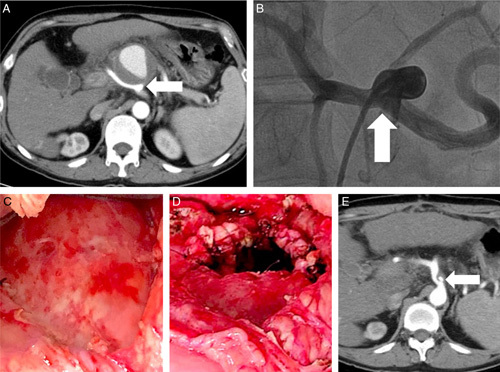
Celiac trunk false aneurysm (A) CT revealed an aneurysm from the celiac trunk (arrow). (B) The angiography showed a false aneurysm about 6 cm in diameter arising from the bifurcation of the celiac trunk(arrow). (C) The false aneurysm was about 7 cm in diameter, and challenging to control the feeding vessels from the outside of the aneurysm due to chronic pancreatitis-related dense adhesion. (D) We opened the aneurysm and directly sutured the bleeder from the inner lumen of the aneurysm. The rim of the false aneurysm cavity was oversewed for hemostasis and the prevention of pancreas leakage. The cavity was packed with an omentum patch to avoid re-accumulated infected fluid. (E) Follow-up CT showed a remaining small false aneurysm 8 mm in diameter (arrow) six months after the surgery.
